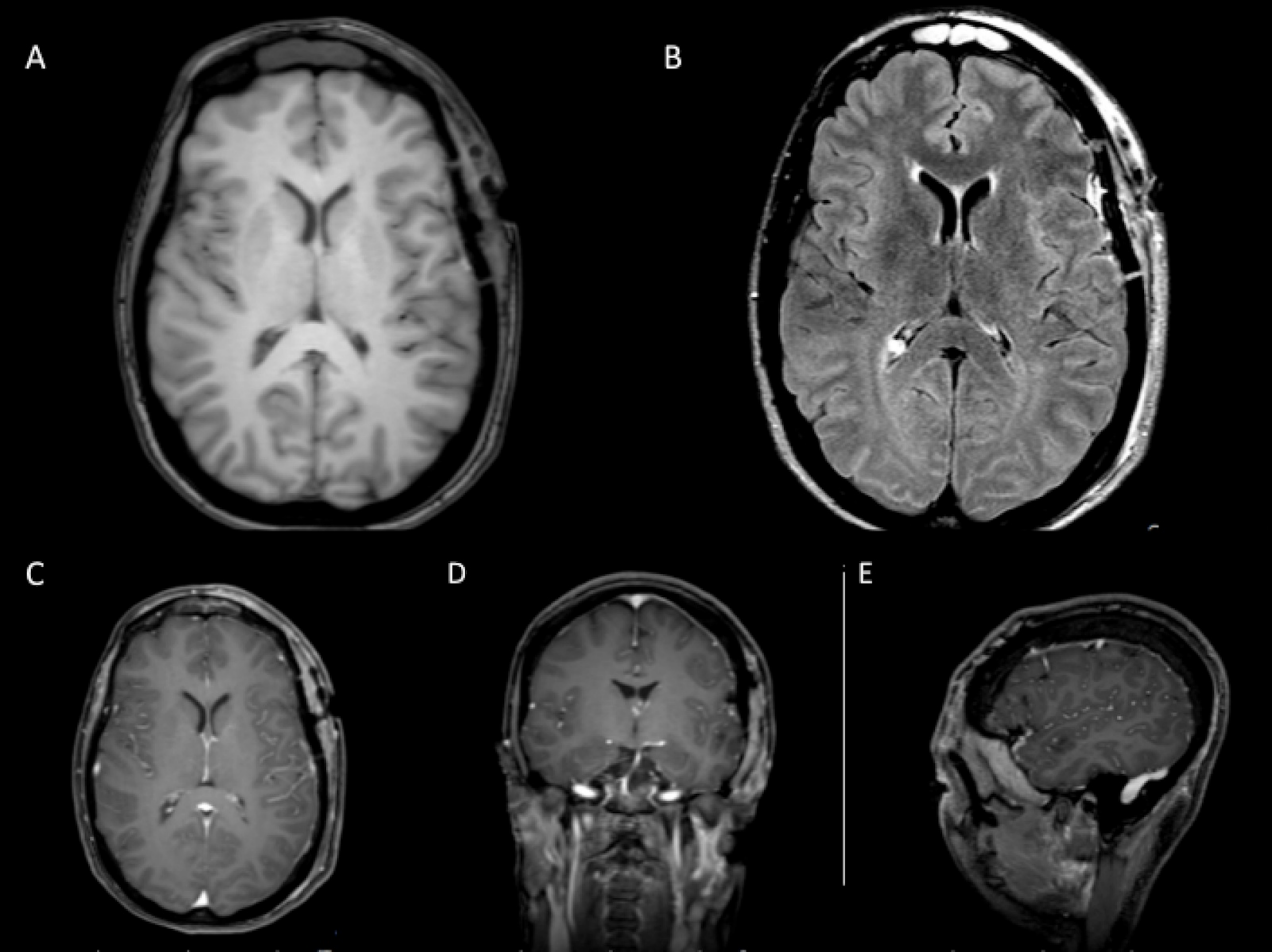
Postoperative imaging for patient two showing gross total resection Magnetic resonance imaging A) non-contrast axial T1, B) axial fluid-attenuated inversion recovery, and April 2017 follow-up C) axial, D) coronal, and E) sagittal T1
